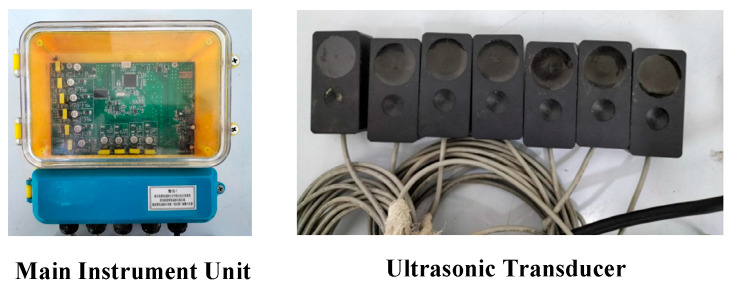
Multi-channel ultrasonic liquid-level interface measurement prototype system.
